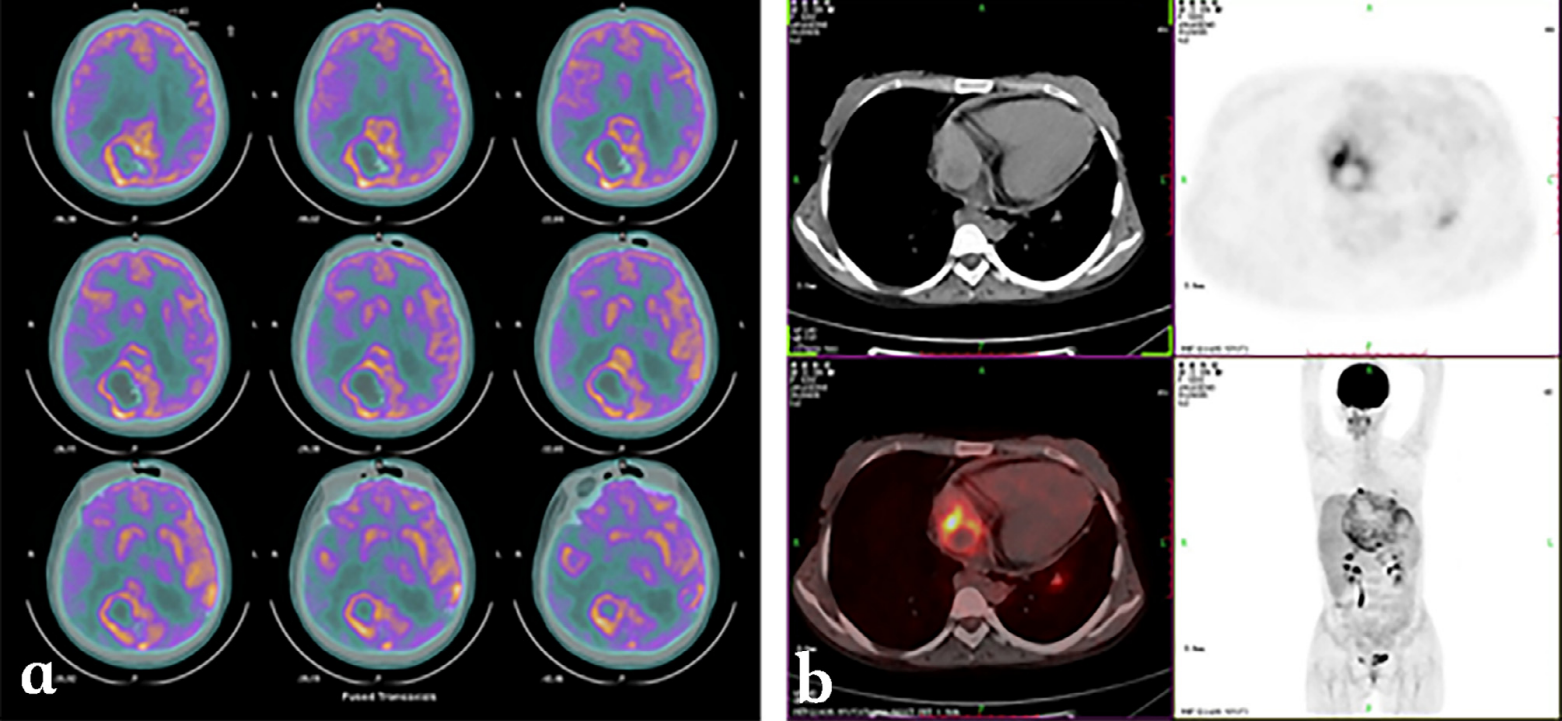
18F-Fluorodeoxyglucose (FDG) Positron-emission tomography (PET) aspects of extrahepatic alveolar echinococcosis.* (a) Brain metastasis of alveolar echinococcosis. (b) Right atrium metastasis of alveolar echinococcosis.
